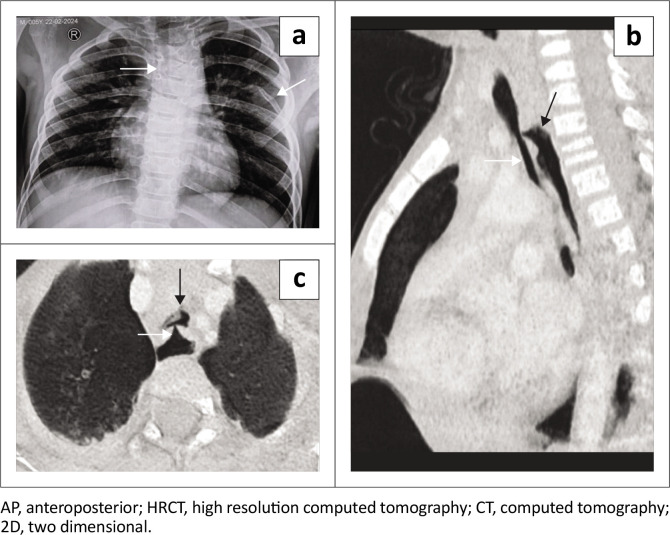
A 3.5-year-old female presented with fast breathing, feeding difficulties and regurgitation of food. (a) AP chest radiograph demonstrates vertebral and rib anomalies (white arrows). Oral swallow study failed to demonstrate the presence of a fistula. Subsequently, HRCT thorax confirmed the presence of an H-type fistula: arrows in (b) sagittal reformatted chest CT image of the trachea (white arrow) and oesophagus (black arrow), and (c) the axial MinIP image of the trachea (black arrow) and fistulous communication (white arrow).
